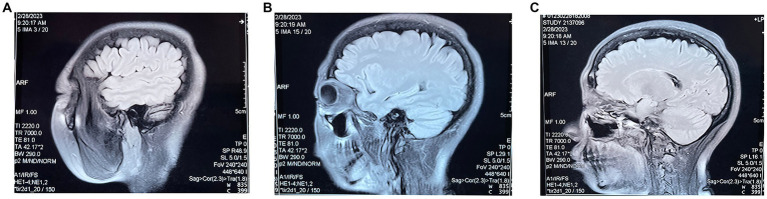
(A–C) are three different sections of the sagittal position of the MRI re-examined 10 months after the patient’s fire needle treatment.
